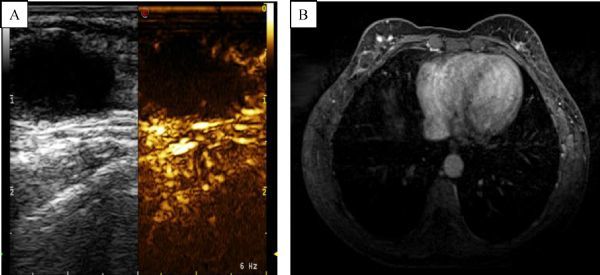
Contrast-enhanced ultrasound results in a patient with a galactocele. A: No obvious enhancement was found; B: In the same patient, enhanced MRI results also showed no significant enhancement.
